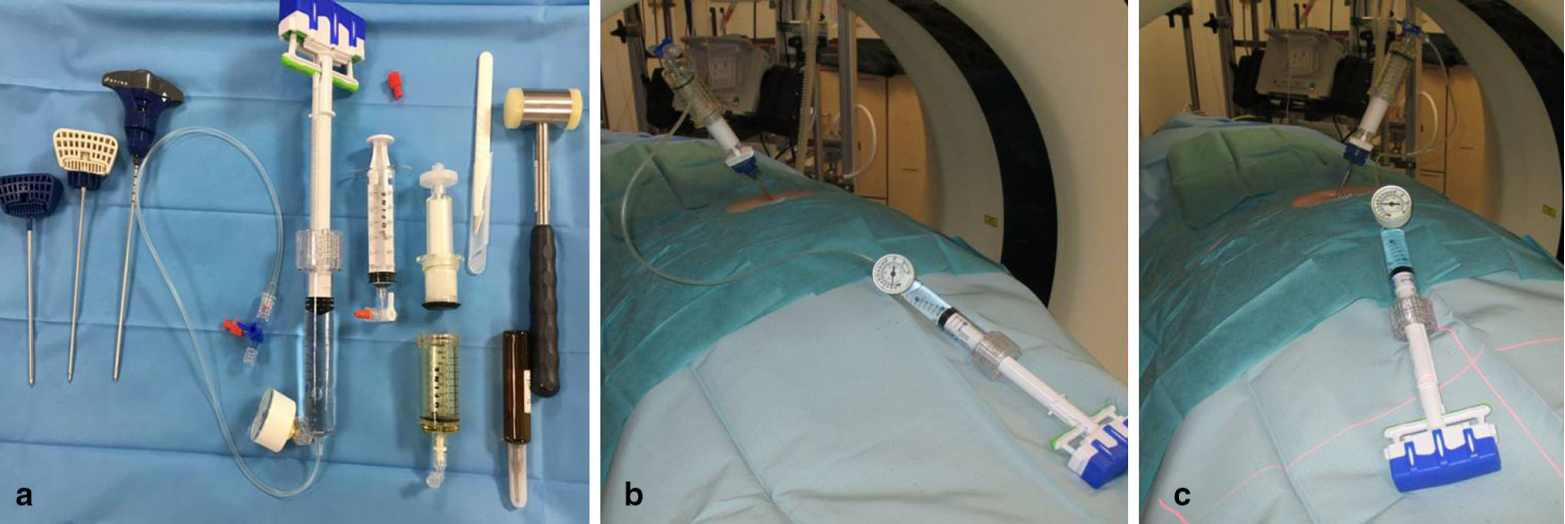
a–c: In a, instruments of the StabiliT^®^ MX Kit plus sterile hammer. In b and c, female patient placed in the prone position in the CT with inserted hollow needle and connected cement applicator with pressure gauge. The approach was made via the short axis in each case, in b to treat the* right side*, in a to treat the* left side*
